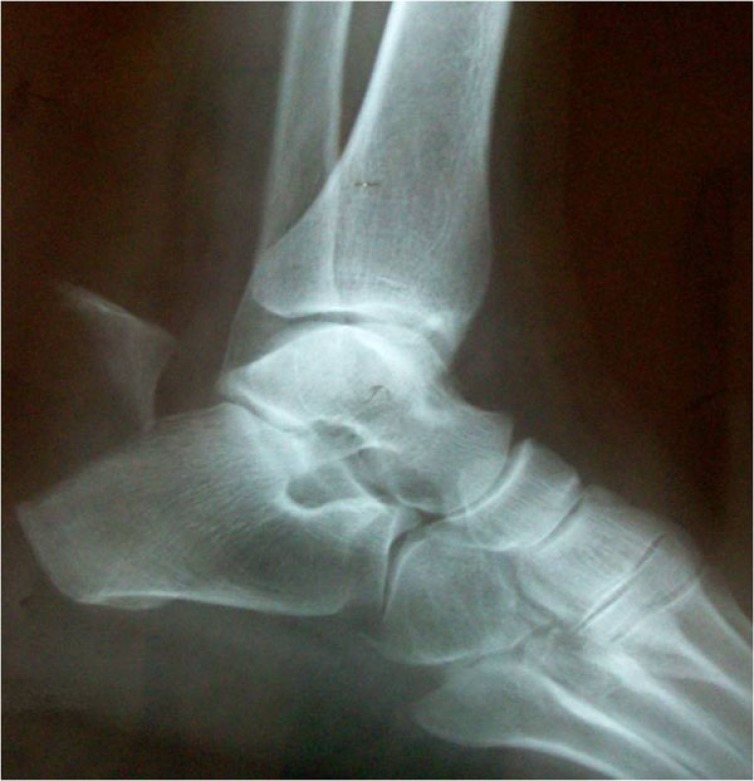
Lateral x-ray of the original fracture.
